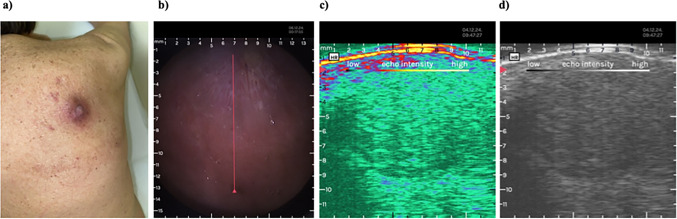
Epidermoid cyst in a 68-year-old male patient, Fitzpatrick skin type II. a Clinical image showing a round, purple-coloured, raised lesion with irregular shape on the left scapular region. The lesion was firm on palpation and moved with the skin. b Dermoscopic image of the lesion. c Dermoscopy-guided high-frequency ultrasound (DG-HFUS) image demonstrating an oval-shaped lesion located at the dermal–subcutaneous border, with a measured depth of 8.7 mm. d DG-HFUS image showing a heterogeneously hyperechoic lesion with regular margins and posterior acoustic enhancement, consistent with an epidermoid cyst. The inhomogeneous hyperechogenicity corresponds to the high keratin content characteristic of epidermoid cysts
